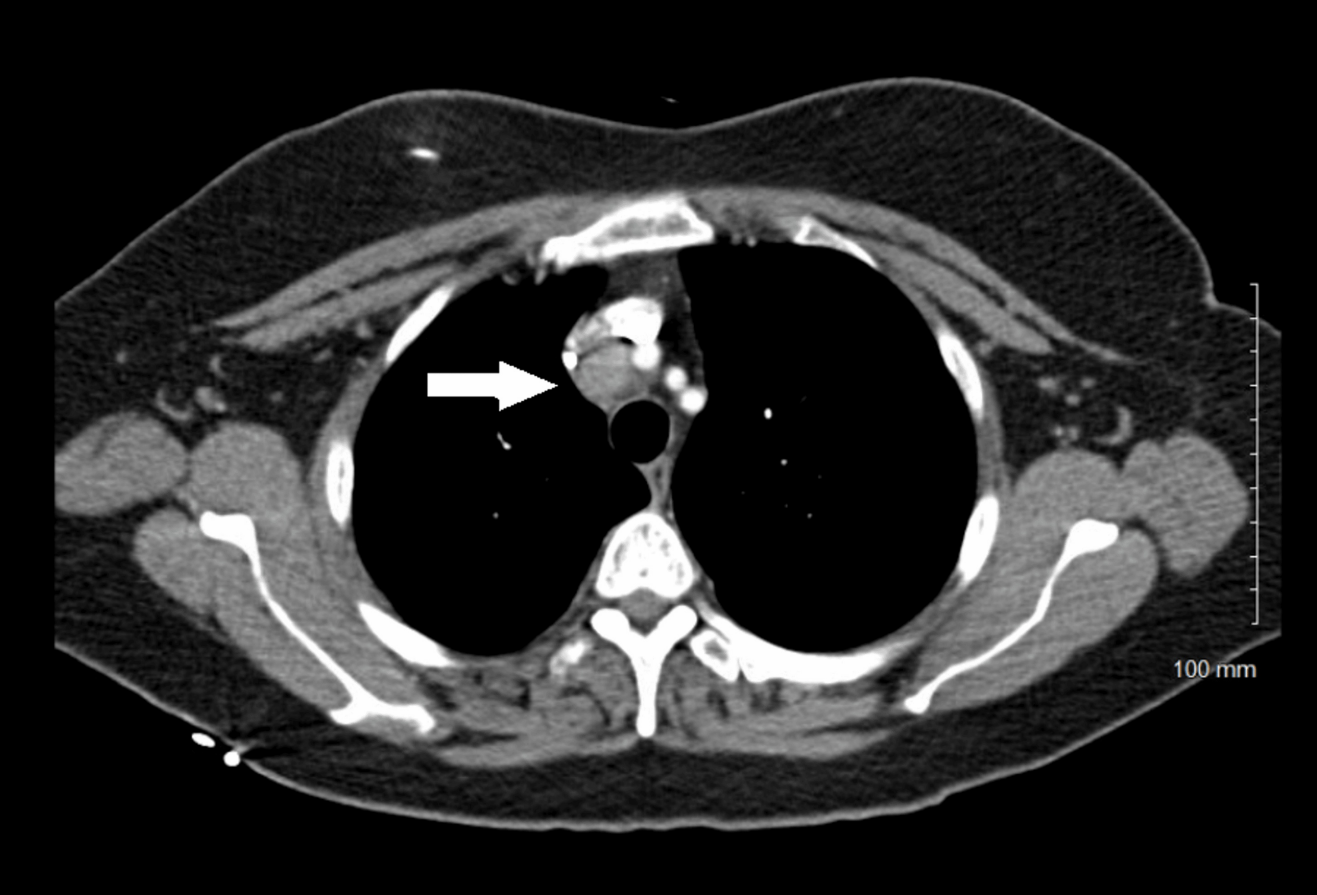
The CT showing metastases in station 2R of the mediastinum (white arrow) CT: computed tomography
